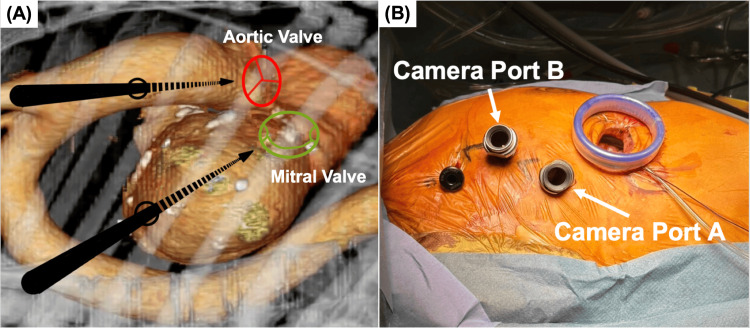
Dual-Camera Port Setup and Intraoperative Port Configuration (A) Preoperative contrast-enhanced computed tomography demonstrating assessment of optimal access trajectories for en face endoscopic visualization of both the aortic and mitral valves. Arrows indicate the estimated camera port entry angles to achieve this view. (B) Intraoperative view of the port configuration, showing a 4-cm main working incision, two camera ports, and an instrument port.
